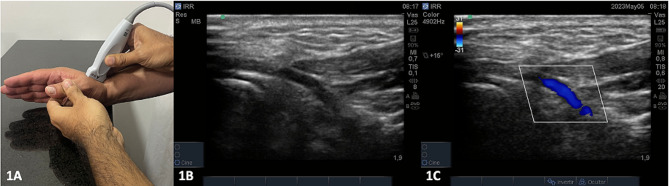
Technique for the measurement of the radial resistance index. A. Transducer placing. B. B Mode examination of the dorsal branch of the radial artery in the anatomical snuffbox. C. Color Doppler mode examination of the dorsal branch of the radial artery in the anatomical snuffbox
